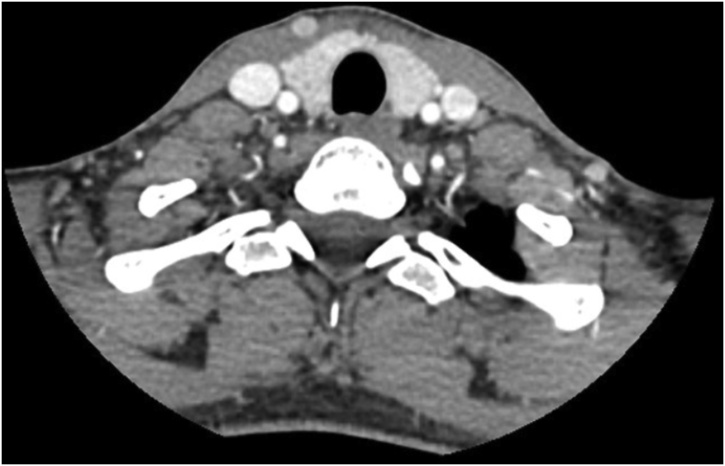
A contrast CT axial scan of the thyroid gland demonstrating no obvious pathology.
